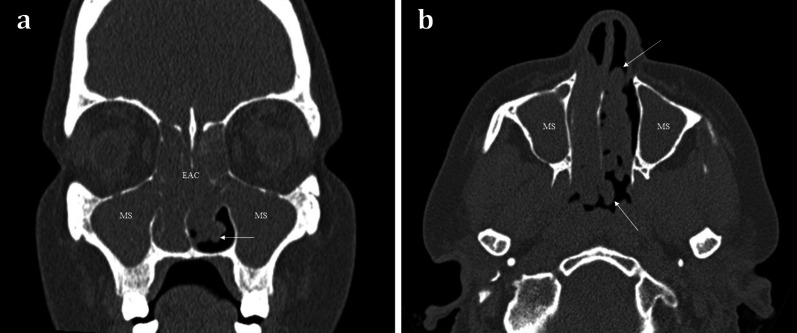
48-year-old female with long standing nasal congestion and rhinorrhoea. Coronal (a) and axial (b) bone window CT images demonstrating extensive sinonasal polyposis with extension into the nasopharynx. Bilateral involvement is suggestive of benign disease. Note the characteristic rounded contours (arrows) which are very suggestive of polyposis. There is also complete opacification of the maxillary sinuses (MS) and ethmoid air cells (EAC)
